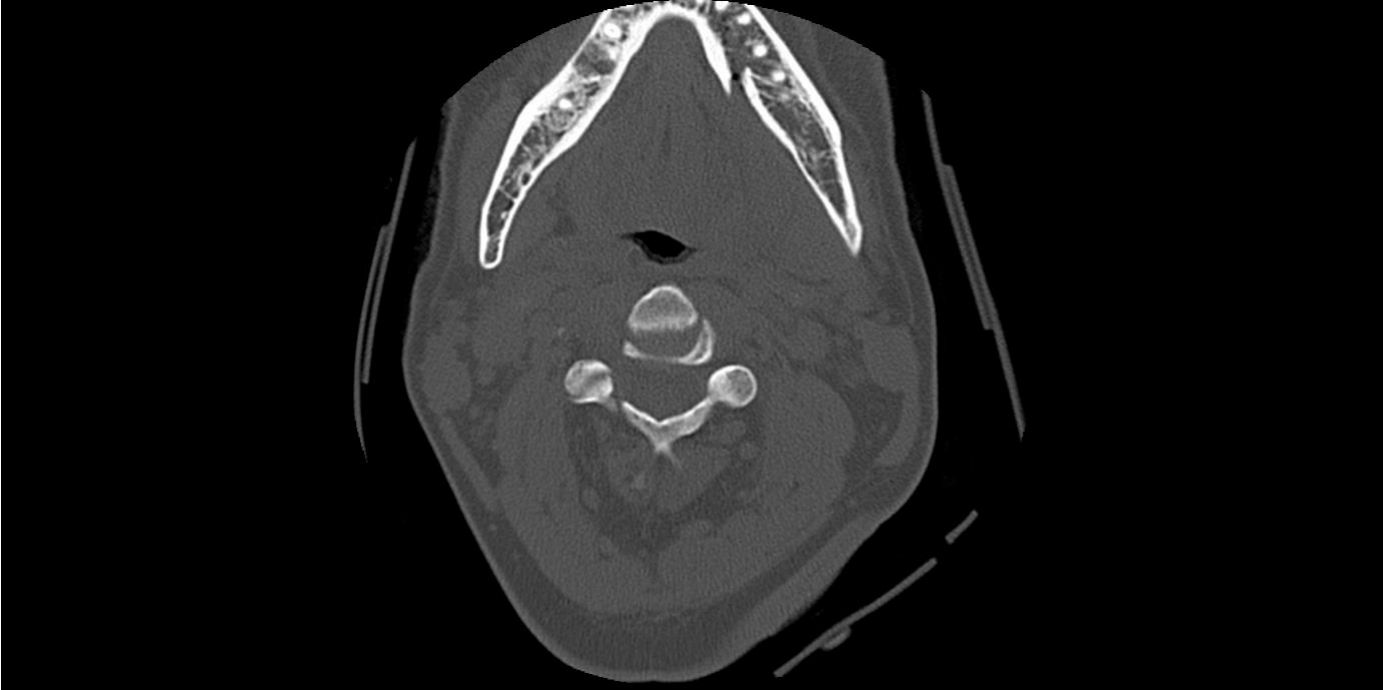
Axial section of computed tomography revealing fracture of mandibular symphysis.
